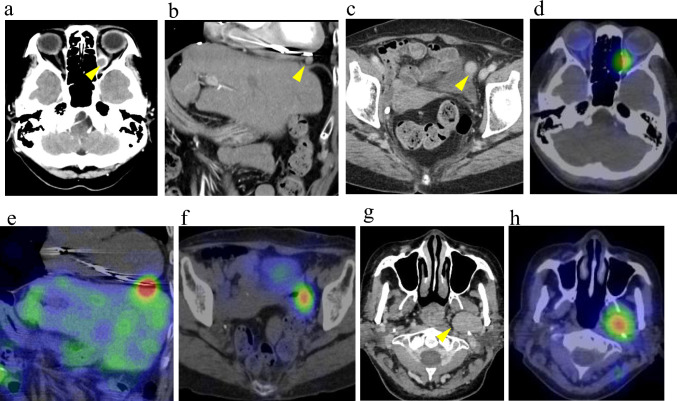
Imaging findings of the systemic lesions. a–c Dynamic CT images: a Head axial view, b chest and abdomen coronal view, and c pelvis axial view. Arrowheads indicate an enhancing mass in the left medial rectus muscle, a small nodule adjacent to the right ventricle, and a faintly enhancing nodule in the left ovary, respectively. d–f SRS: Images correspond to sections (a), (b), and (c). Each lesion identified on CT shows somatostatin receptor expression. g Enhanced head CT (axial view): The arrowhead indicates a known pleomorphic adenoma. h SRS (head, axial view): Significant uptake is noted in the pleomorphic adenoma, corresponding to the CT finding in g
